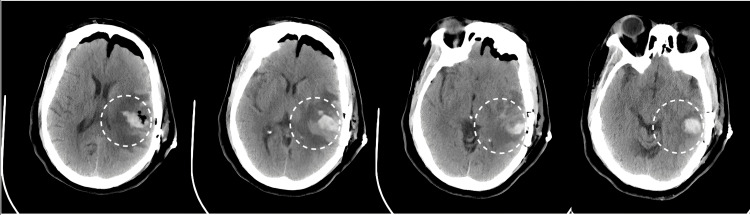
Progressive axial CT scans after the patient demonstrated a postoperative change on the neurological examination.
